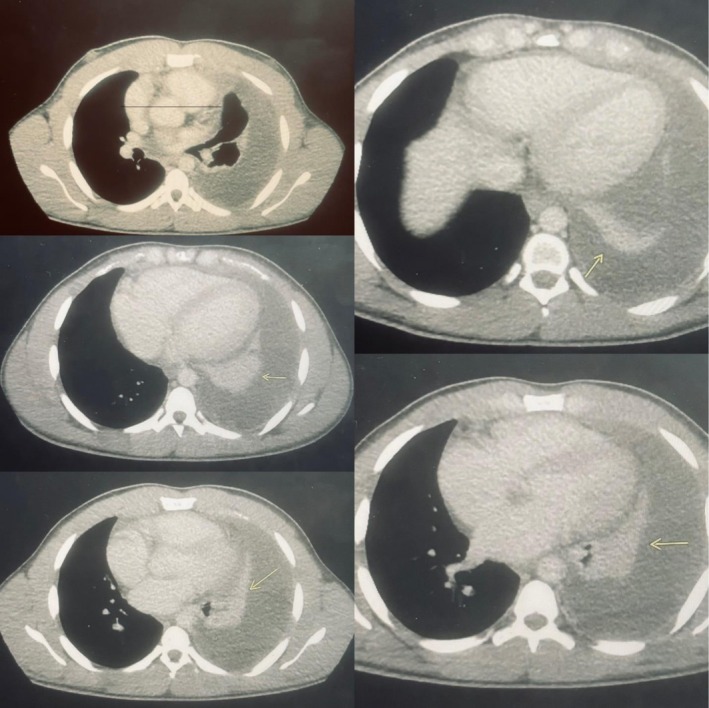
Computed tomography (CT) scan of the chest with contrast, with a transverse line showing a borderline enlarged heart, and additional images, including views of the lung bases with yellow arrows showing a large left pleural effusion, loss of upper left lung volume indicating collapsed portions of the left upper lobe, and a compression atelectasis involving much of the left lower lobe.
